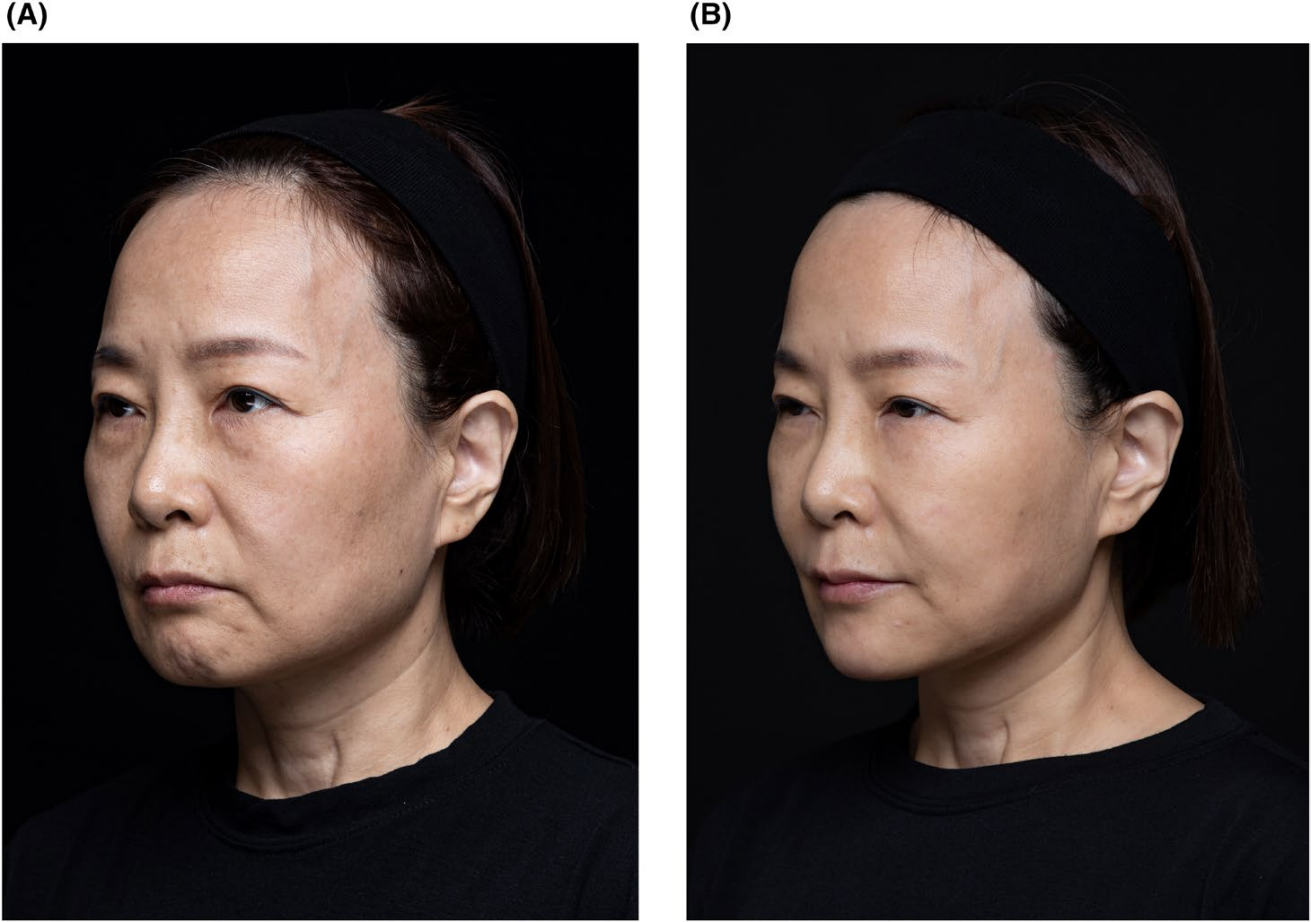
Use of EBD and VYC‐12L within the same treatment session. This 52‐year‐old woman was treated with two different types of EBD (microfocused ultrasound and radiofrequency; full face), Juvéderm fillers (VYC‐20L, 4.5 mL per side in the temples, cheeks, chin, and jaw; VYC‐17.5L, 2 mL per side in the nasolabial folds and lips; VYC‐15L, 0.5 mL per side in the tear troughs), as well as VYC‐12L (cheeks, 2 mL per side), all within the same session. The images show her before treatment (A), and 1‐month post‐treatment (B). The impact of adding VYC‐12L is particularly evident in improved skin texture and reduced roughness. Images are courtesy of Wonseok Choi. EBD, energy‐based device.
